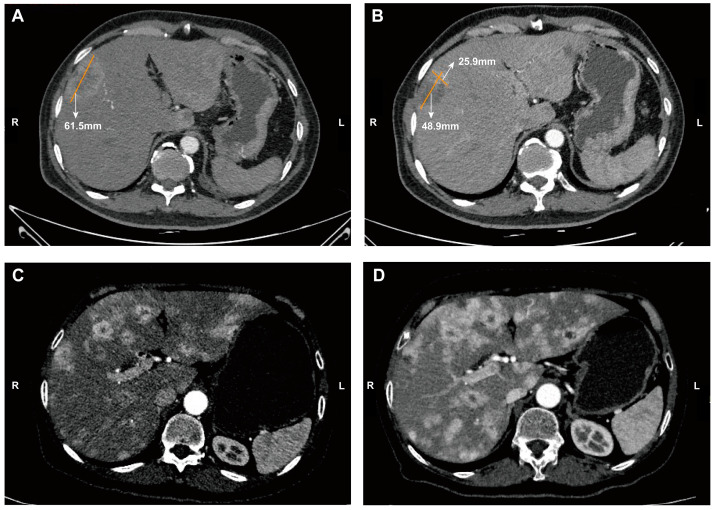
(A) Baseline CT image from a 56-year-old male patient with liver metastases of a WHO Grade 3 pancreatic neuroendocrine tumor. The longest diameter of one target lesion of liver metastasis was 61.5 mm. (B) After two procedures of TAE treatment, the longest diameter of this target lesion decreased to 48.9 mm. The longest diameter of the enhanced tumor region decreased to 25.9 mm. The general tumor response assessment for this patient was SD using RECIST criteria but partial response using mRECIST criteria. (C) Baseline CT image of liver metastases from a 66-year-old female patient with liver metastases with a WHO Grade 2 pancreatic neuroendocrine tumor. (D) After one procedure of TAE treatment, the patient had PD.
